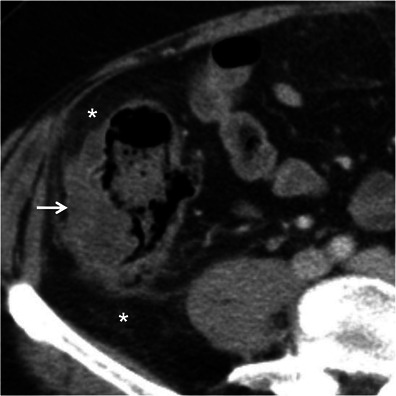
Colon cancer. Axial contrast-enhanced CT scan shows focal asymmetric and irregular thickening of the ascending colon (*arrow*), a finding strongly suggestive of a neoplasm. Also note mild pericolonic fat stranding (*asterisks*), a frequent associated finding. *Adapted from the electronic poster “Bowel wall thickening—a complex subject made simple” DOI:*10.5444/esgar2011/EE-063
